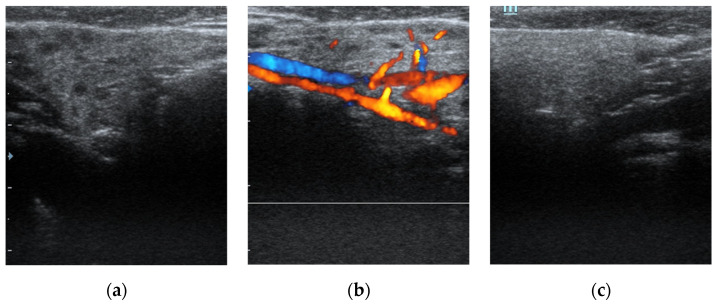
Ultrasound diagnostics of juvenile recurrent parotitis. (a) displays a unilaterally affected parotid gland on the right side of a patient. Note heterogeneous parenchyma with several hypoechoic nodules indicating duct dilatation. (b) demonstrates increased blood flow of the same parotid gland with Doppler mode. In (c), a normal parotid gland on the left side of the same infant is shown as a comparison.
